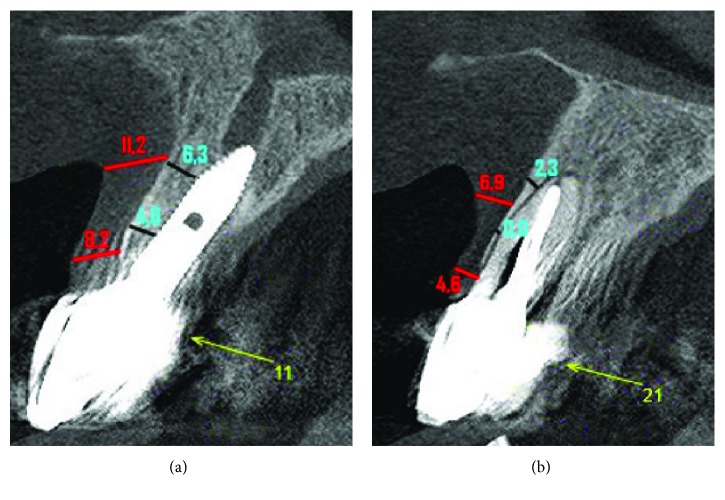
CBCT scan of the follow-up of the IDR reapplication. (a) Tomography with measures of bone and gingival tissue in the vestibule of implant 11 which was reconstructed by the surgical procedures. (b) Tomography where it is possible to compare the volumes of bone and gingival tissue in the vestibular area of a healthy tooth 21.
